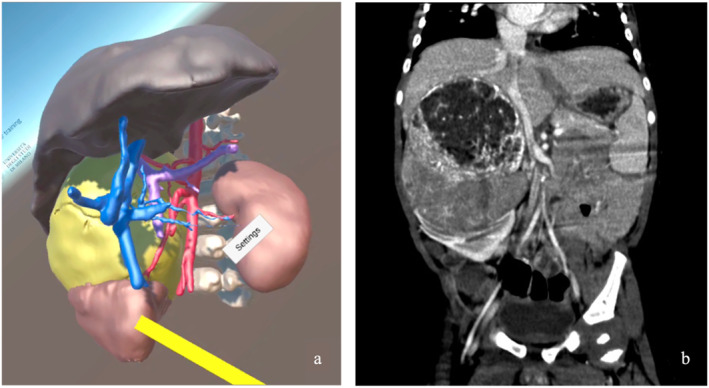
Multimodal representation of the right adrenal neuroblastoma: (a) VR reconstructed anatomical visualization showing the tumor's spatial relationship with adjacent organs and artery distribution, highlighted with a yellow marker and (b) coronal CT scan demonstrating the large abdominal mass with heterogeneous density, indicative of its complex nature and involvement with surrounding tissues.
